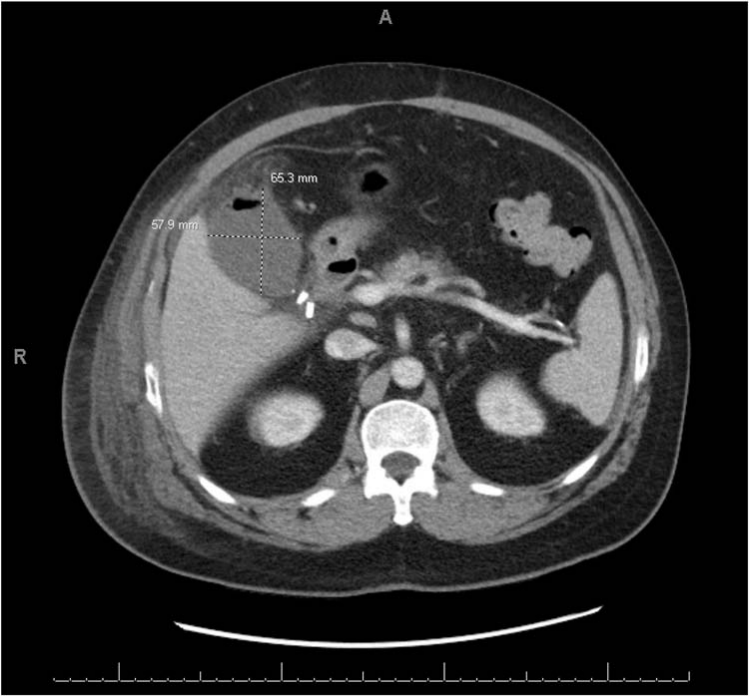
CT abdomen and pelvis with IV contrast demonstrating a complex fluid density with gaseous foci occupying the gallbladder fossa and measuring 6.5 cm × 5.8 cm × 5.5 cm.
